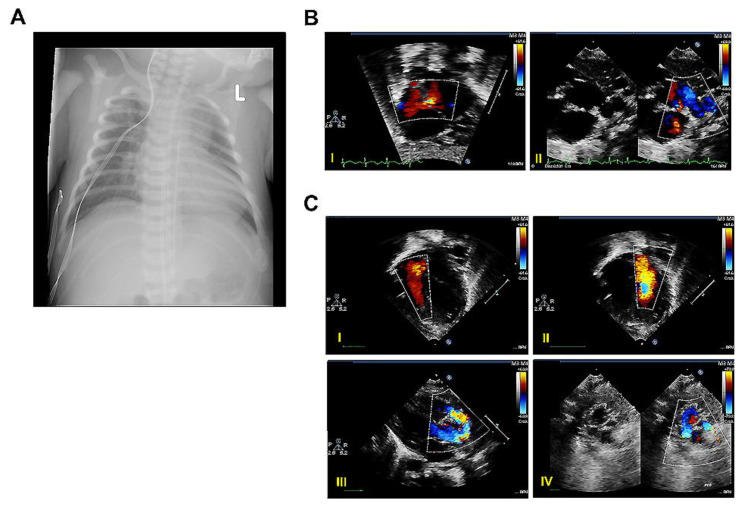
Clinical features of proband B. (A) Representative chest X-ray on day of life 0 (DOL 0) showing cardiomegaly. L: Left. (B) Representative echocardiography images on DOL 0. (I) Color Doppler image across the intra-atrial communication after atrial balloon septoplasty; (II) Color Doppler image showing patent ductus arteriosus. (C) Representative echocardiography images on DOL 6. (I,II) Color Doppler images across the tricuspid valve (I) and the mitral valve (II); (III) Color Doppler image of aortic arch after arterial switch operation; (IV) B-mode and color doppler images of branch pulmonary arteries after arterial switch operation.
